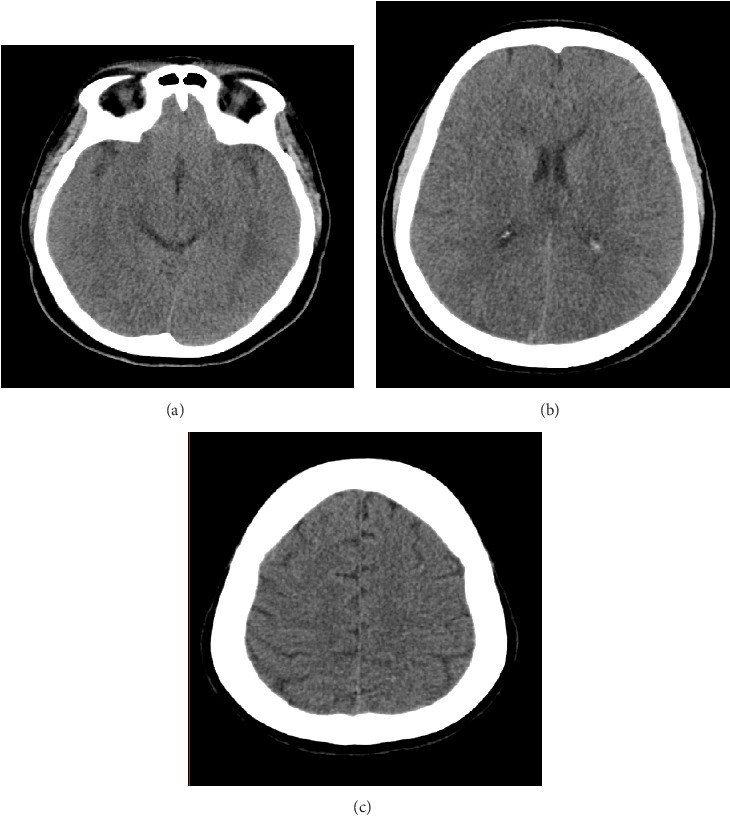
Sections of the patient's head CT scan without any significant findings.
